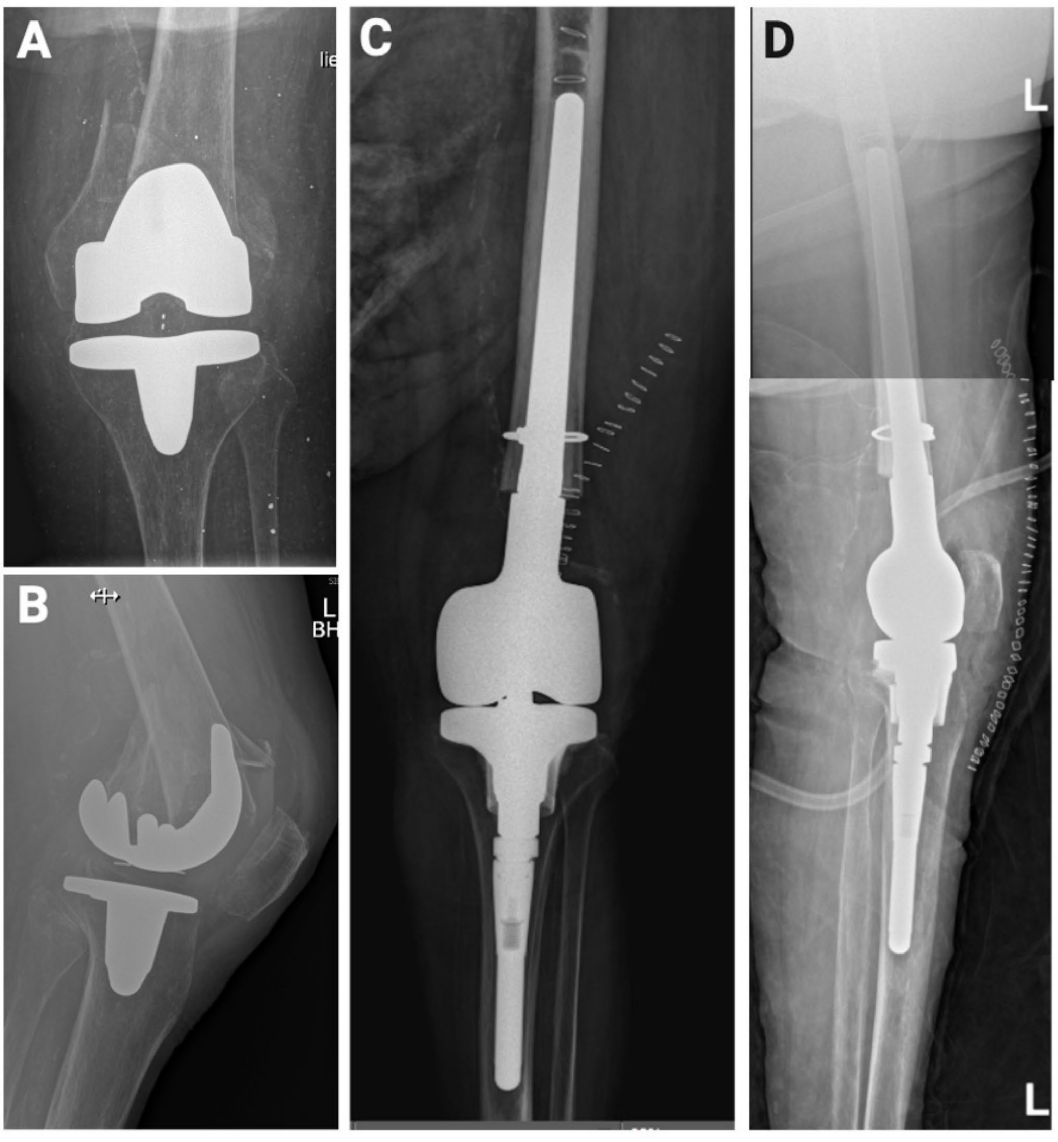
Case example of a Lewis–Rorabeck Type III periprosthetic distal femur fracture. Pre- and postoperative radiographs of an 84-year-old female patient with a Lewis–Rorabeck Type III periprosthetic distal femur fracture sustained after a fall from standing height. A Preoperative anteroposterior and B lateral radiographs of the knee demonstrating the fracture. C Postoperative anteroposterior and D lateral radiographs following distal femoral replacement, performed the day after hospital admission
